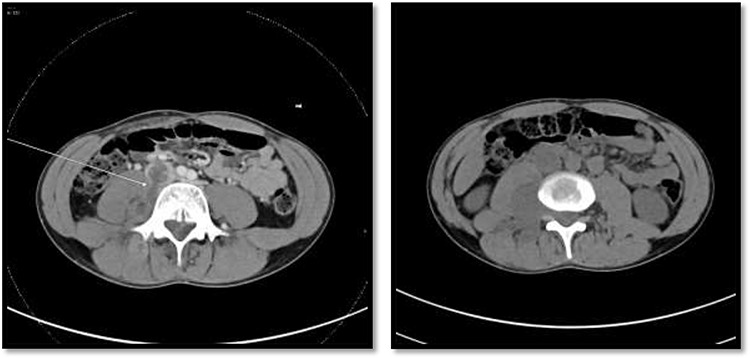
On the left image, there is associated fistulas tract between the mass and infrarenal inferior vena cava with a small hyperdense component may represent hemorrhage or solid component. The right image CT scan showed the mass extending through the neural foramina compressing the spinal cord from the level of the L2–L4.
